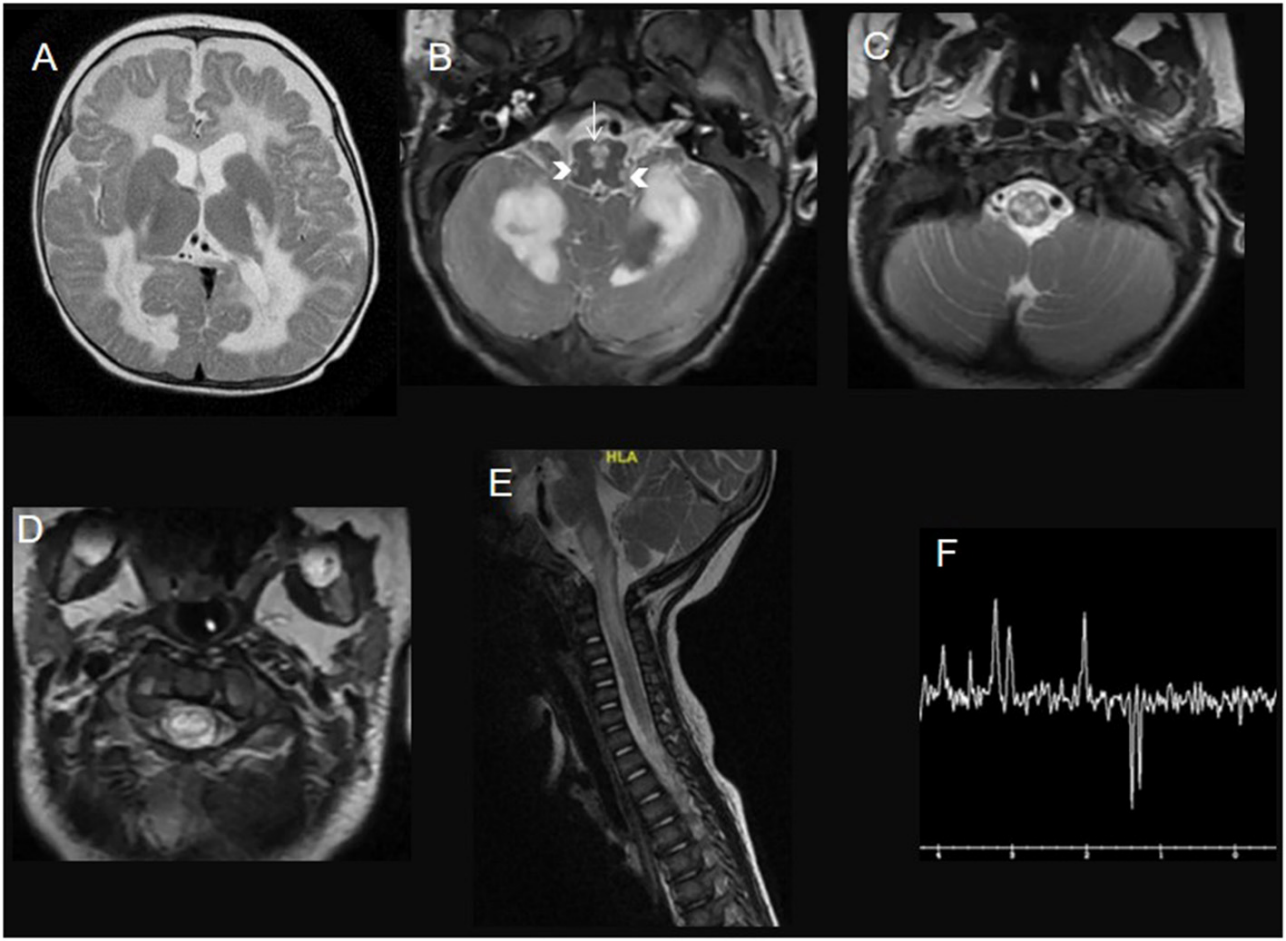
A 3-year-old child with leukoencephalopathy with the brainstem, spinal cord involvement, and lactate elevation due to *DARS2* mutation. Brain (A–C) and spinal cord (D,E) MRI T2WI with Long Echotime MRS (F) hyperintensity of the deep white matter tracts in the brain (involving the posterior limb of internal capsules and sparing the subcortical U fibers), the deep cerebellar white matter, the pyramids in the medulla (white arrow) and the inferior cerebellar peduncles (head arrows), the dorsal column and lateral corticospinal tracts over the rostral spinal cord. Long echo time MRS (F) shows an inverted prominent lactate peak at 144 ms.
